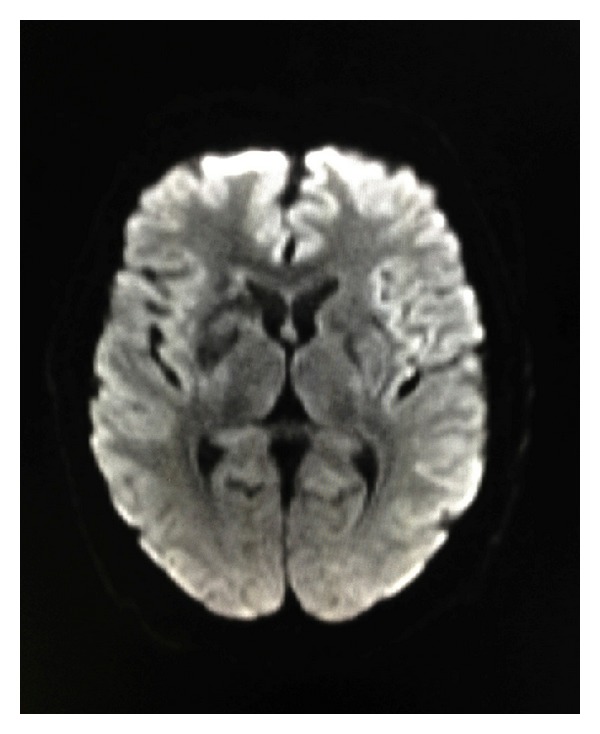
Axial DWI does not show evidence of restricted diffusion.
